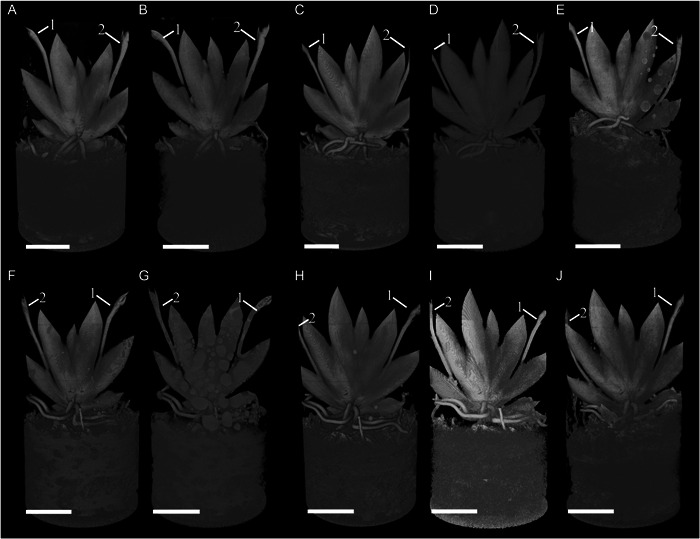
Screen recordings of two sides of a Falcon container containing a flowering individual of *Erycina pusilla*, which was repeatedly scanned using micro‐CT during a 19‐week period. (A–E) Front side after the first week (A), second week (B), fifth week (C), 12th week (D), and 19th week (E) of scanning. (F–J) Back side after the first week (F), second week (G), fifth week (H), 12th week (I), and 19th week (J) of scanning. Different inflorescence stalks are labeled with numbers 1 and 2. Scale bar = 1 cm.
